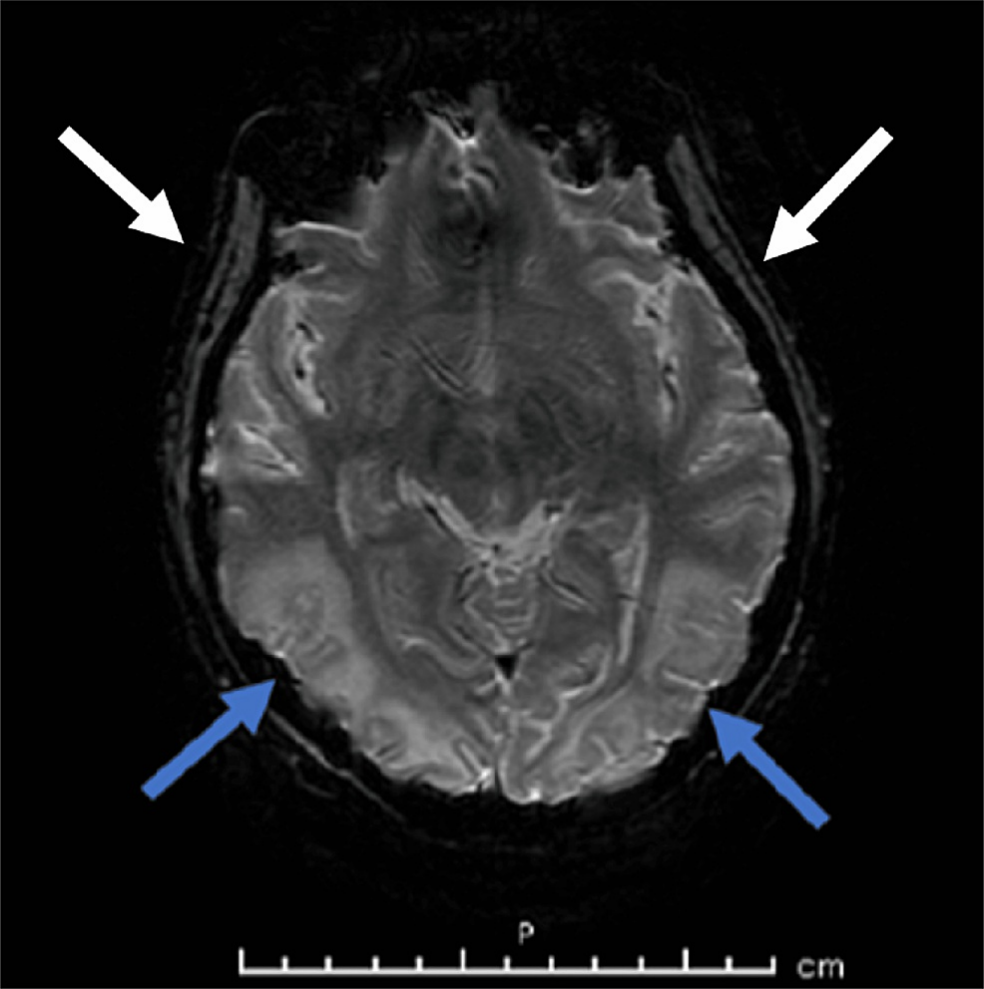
Non-contrast MRI of the brain with posterior (blue arrows) and frontoparietal (white arrows) extensive bilateral cerebral and cerebellar deep white matter high-intensity signals.
